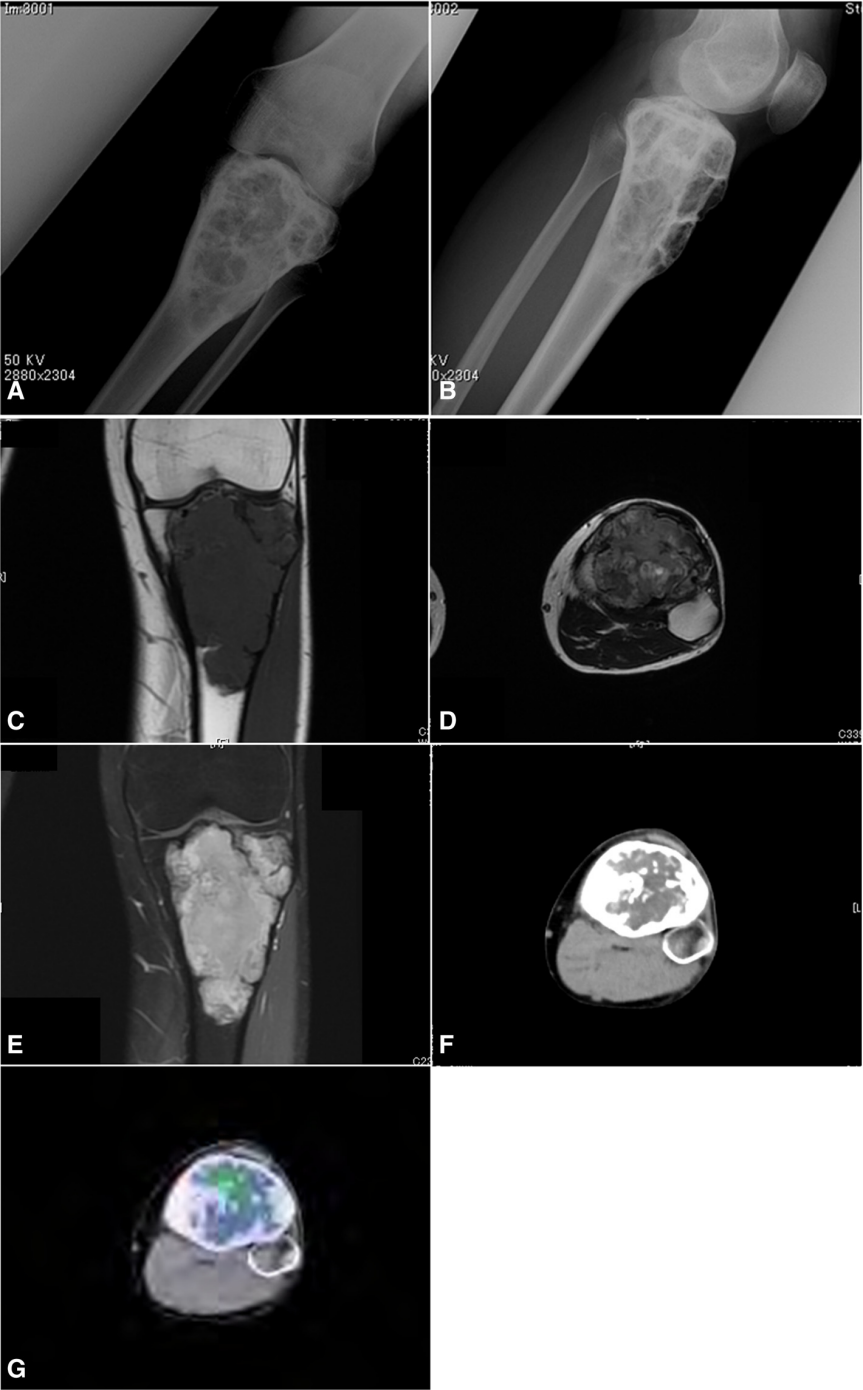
Post-treatment radiological analyses of the left knee. Plain radiographs show marked osteosclerosis and sclerotic rim formation on both the proximal tibial tumor (A, B) and distal femoral tumors. MRI shows a tumor displaying iso-intensity to the surrounding muscle on T1-weighted imaging (coronal view) (C), heterogeneous high intensity (but lower than at pretreatment) on T2-weighted imaging (axial view) (D), and diffuse enhancement on gadolinium-enhanced T1-weighted fat-suppression imaging (coronal view) (E). ^18^F-FDG PET/CT revealed the marginal/intralesional osteosclerosis of the proximal tibial tumor (F) with markedly decreased SUV uptake (SUVmax: 4.8 in the tibial tumor) (G).
